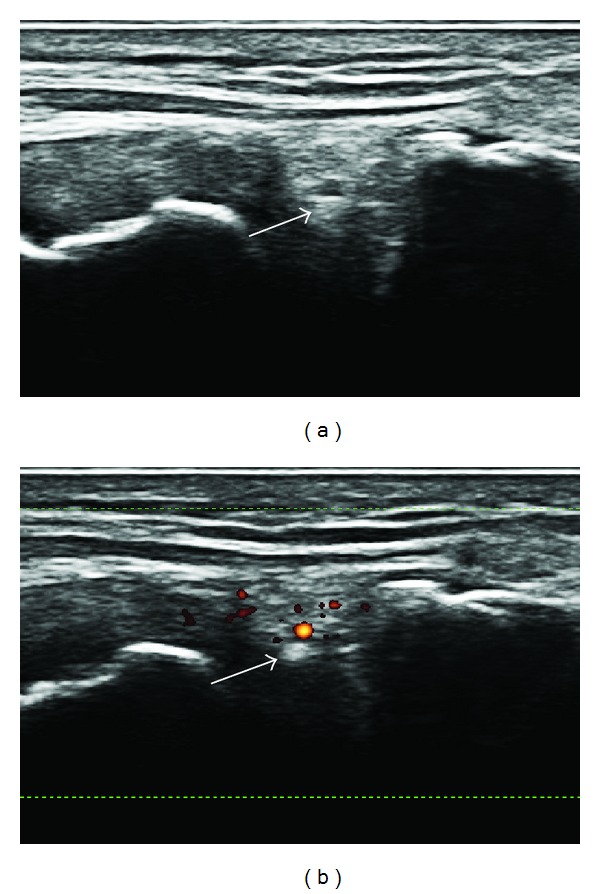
Small hyperechogenic deposit in a medial meniscus (arrow). Power Doppler US highlights the presence of a small vessel just above the focal hyperechogenicity, stressing the probability of posterior enhancement due to the presence of blood (water) in the vessel.
